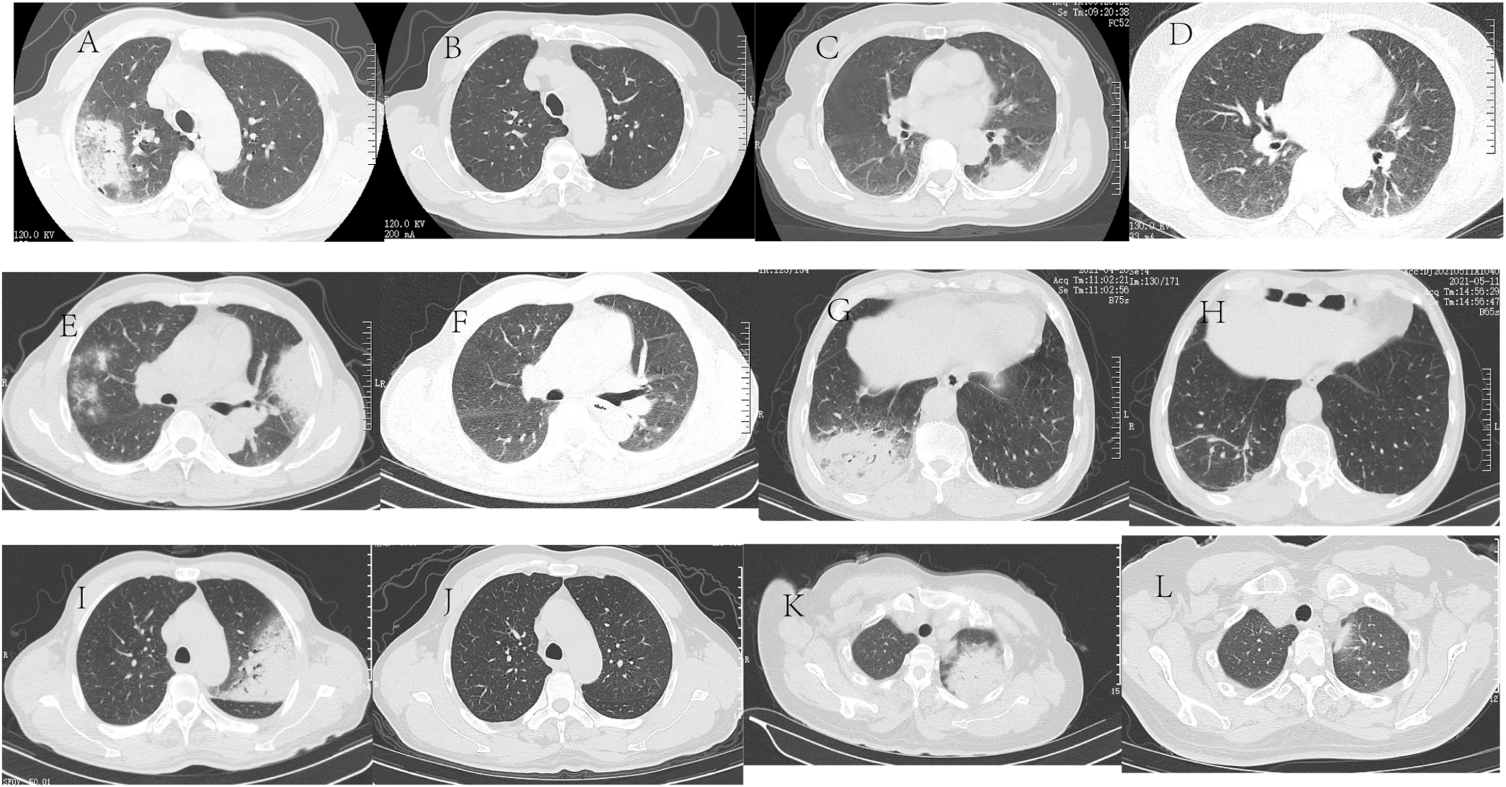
Chest CT of six patients with *C psittaci* pneumonia Chest CT of six patients with *C psittaci* pneumonia. Case 1: A 68-year-old male was admitted because of a fever and headache for three days. (A) Chest CT shows a patchy high-density shadow subpleural region in the right upper lung, with a small amount of pleural effusion in the right pleural cavity. (B) Two months after discharge from the hospital, chest CT showed that the inflammation in the upper lobe of the right lung almost disappeared. Case 2: A 69-year-old female was admitted because of a fever for two days. (C) Chest CT shows patchy and mass high-density shadow in the upper and lower lobes of the left lung, with a sign of an air bronchogram. (D) Two months after discharge from the hospital, chest CT showed that the lesions in the upper and lower lobes of the left lung almost disappeared. Case 3, A 51-year-old male was admitted because of left-sided chest pain with a fever for three days. (E) Chest CT shows bilateral multiple patchy and mass high-density shadows, accompanied by local consolidation and air bronchogram sign with a small amount of bilateral pleural effusion. (F) One month after discharge from the hospital, chest CT showed that the inflammation in the bilateral lungs disappeared. Case 4: A 69-year-old male was admitted because of a fever for seven days. (G) Chest CT shows a patchy high-density shadow in the lower lobe of the right lung, with local consolidation and air bronchogram sign. (H) One month after discharge from the hospital, chest CT showed that the inflammation in the lower lobe of the right lung disappeared. Case 5: A 63-year-old male was admitted because of a cough and fever for ten days. (I) Chest CT shows a large patchy high-density shadow in the left upper lung, with local consolidation; A few consolidation shadows in the left lower lung and a small amount of fluid in the left pleural cavity. (J) Two months after discharge from the hospital, chest CT showed that the inflammation in the upper lobe of the left lung almost disappeared. Case 6<nbsp/>A 58-year-old female was admitted because of a fever for four days. (K) Chest CT shows a mass high-density shadow in the left upper lung, with consolidation and air bronchogram sign. (L) One month after discharge from the hospital, chest CT showed that the inflammation in the upper lobe of the left lung disappeared.
